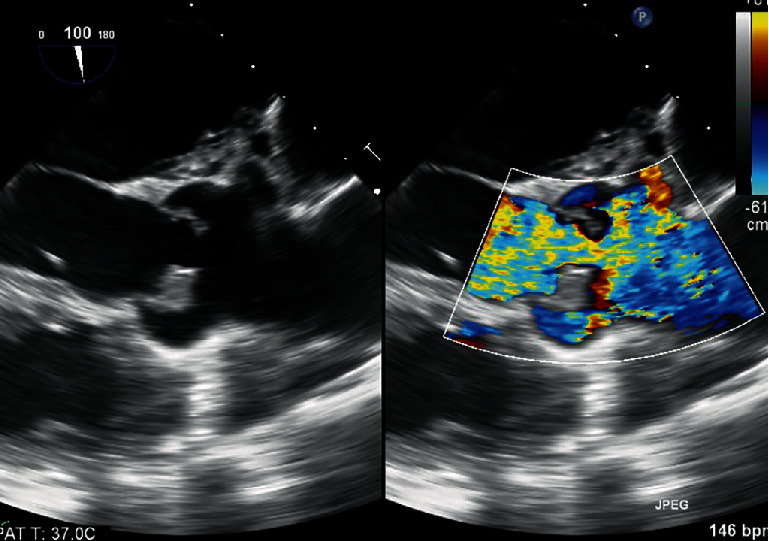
TEE from transgastric long axis view with beam path at 120 degrees showing aortic valve echodensity. Color Doppler demonstrates severe AI. Yellow arrow points to subaortic membrane.
